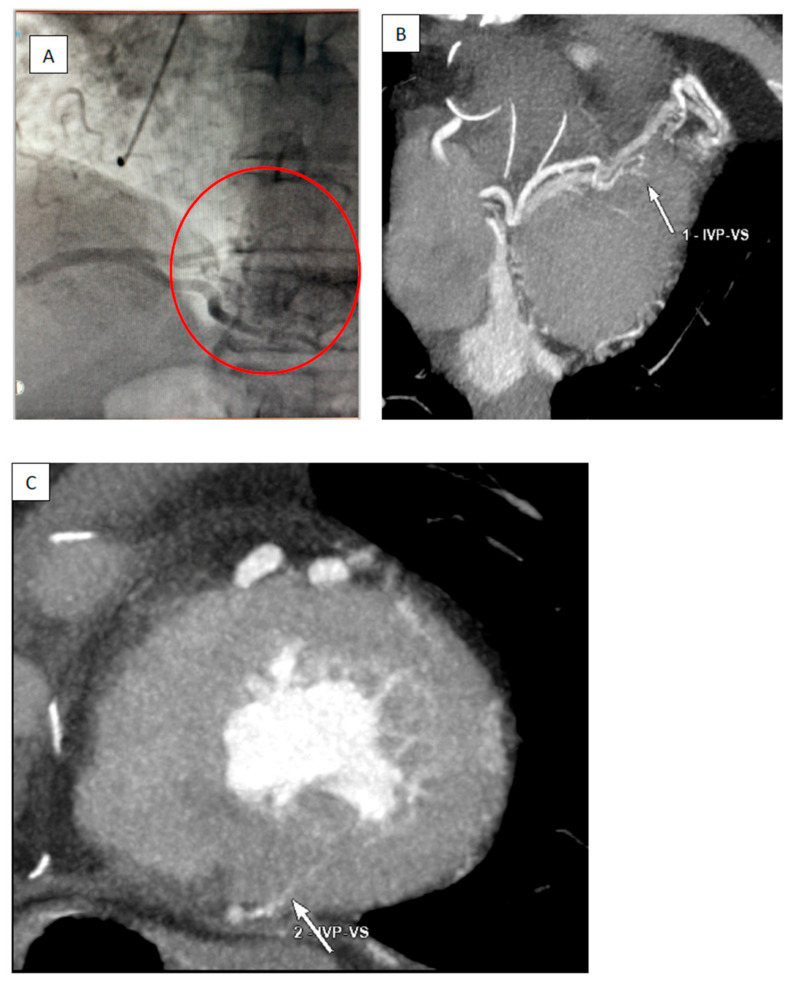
(A) Coronary angiography showing draining of the coronary contrast from the posterior interventricular artery into the left ventricle (circle insert). (B,C) Contrast chest CT showing coronary cameral fistula between posterior interventricular artery (IVP) and left ventricle (VS).
